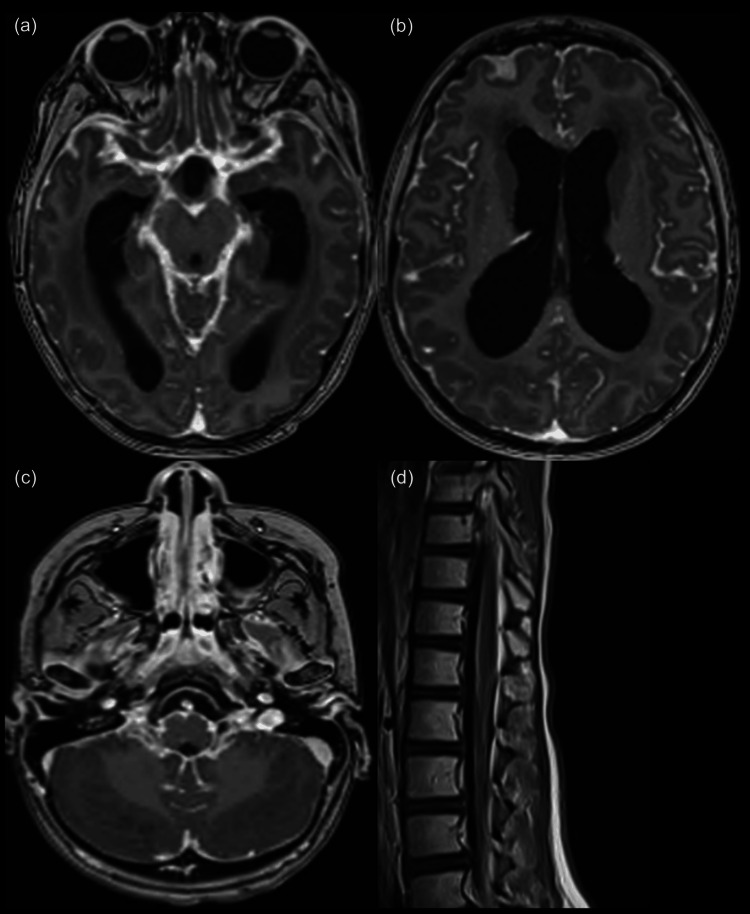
(a-d) MRI brain and spine showing extensively progressive disease both intracranial and intraspinal with stable ventriculomegaly
